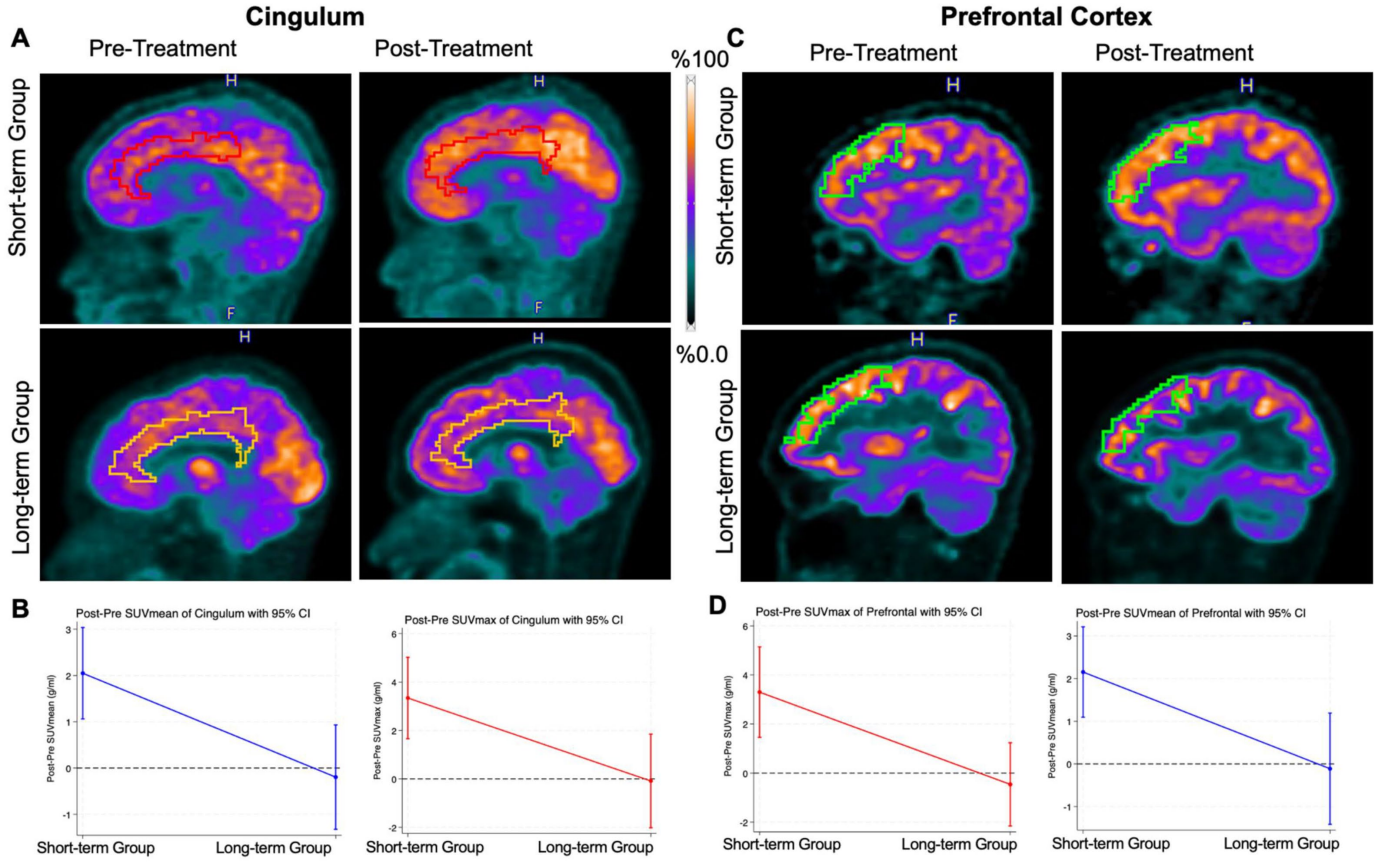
Representative PET images and quantification of FDG uptake in the cingulum and prefrontal cortex. (A) Representative ^18^F-FDG PET scans of a pediatric cancer survivor before HDMTX therapy at 39 days after completion of HDMTX (top row) demonstrates increased FDG uptake of the cingulum on the post-treatment scan compared to the pre-treatment scan. And, ^18^F-FDG PET scans of a pediatric cancer survivor demonstrates no significant change in FDG uptake of the cingulum before HDMTX therapy and at 371 days after completion of HDMTX (below row). (B) Differences between baseline and post-treatment SUV values of the cingulum at 1–3 months (short-term follow up, *n* = 11) and > 3 months (long term follow up, *n* = 8). Generalized linear regression model. Error bars represent the 95% confidence intervals. (C) Representative ^18^F-FDG PET scans of a pediatric cancer survivor before HDMTX therapy at 39 days after completion of HDMTX (top row) demonstrates increased FDG uptake of the prefrontal on the post-treatment scan compared to the pre-treatment scan. And,18F-FDG PET scans of a pediatric cancer survivor demonstrates no significant change in FDG uptake of the prefrontal before HDMTX therapy and at 246 days after completion of HDMTX (below row). (D) Differences between baseline and post-treatment SUV values of the prefrontal at 1–3 months (short-term follow up, *n* = 11) and > 3 months (long term follow up, *n* = 8). Generalized linear regression model. Error bars represent the 95% confidence intervals.
